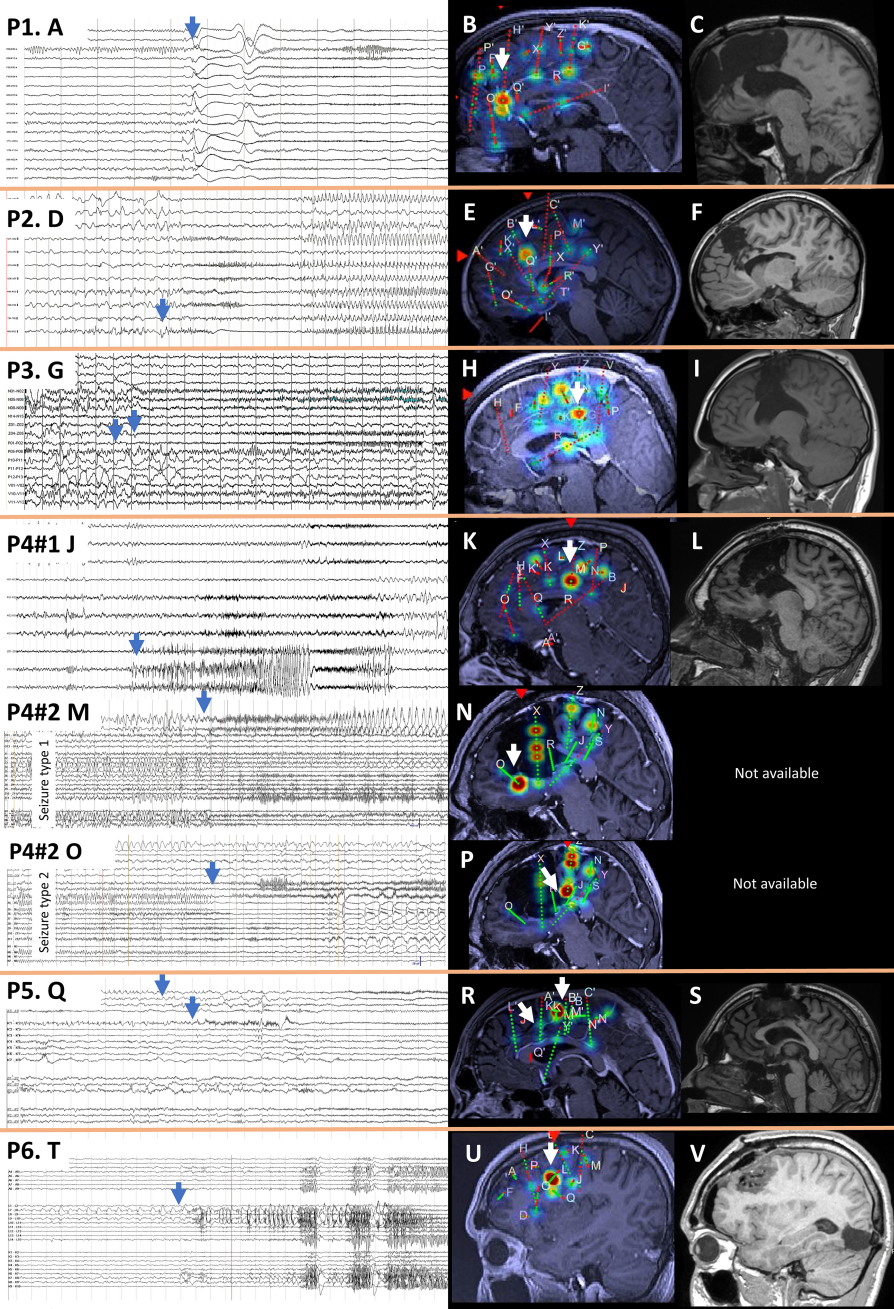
Intracranial electrophysiology, ictal activity maps in the gamma band (patients 1–3) or instantaneous weighted power ratio (IWPR) (patients 4–6) and postsurgical magnetic resonance imaging (MRI) for each patient. We have marked the electrical onset on stereoelectroencephalography traces with a blue arrow (A, D, G, J, M, O, Q, T) and the corresponding brain regions are marked by a white arrow (B, E, H, K, N, P, R, U). Brain regions that display fast activity at seizure onset as shown by gamma and IWPR (displayed using red and orange plots over the patients MRI) were included in the resection (C, F, I, L, S, V).
